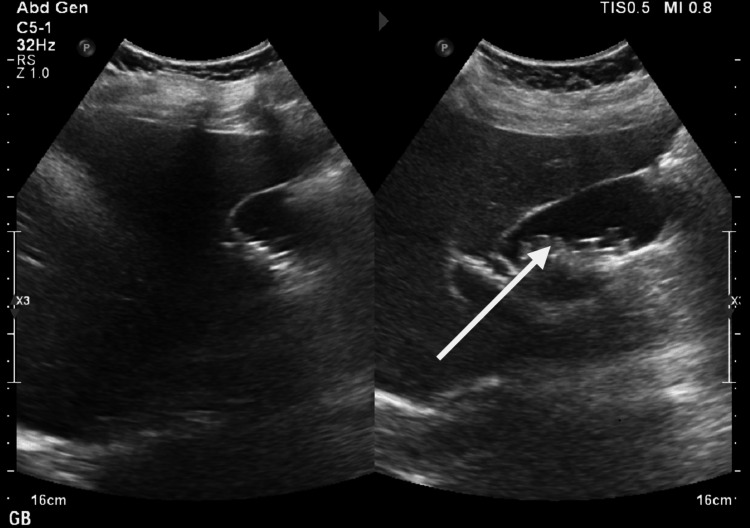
Ultrasound showing multiple gall stones
